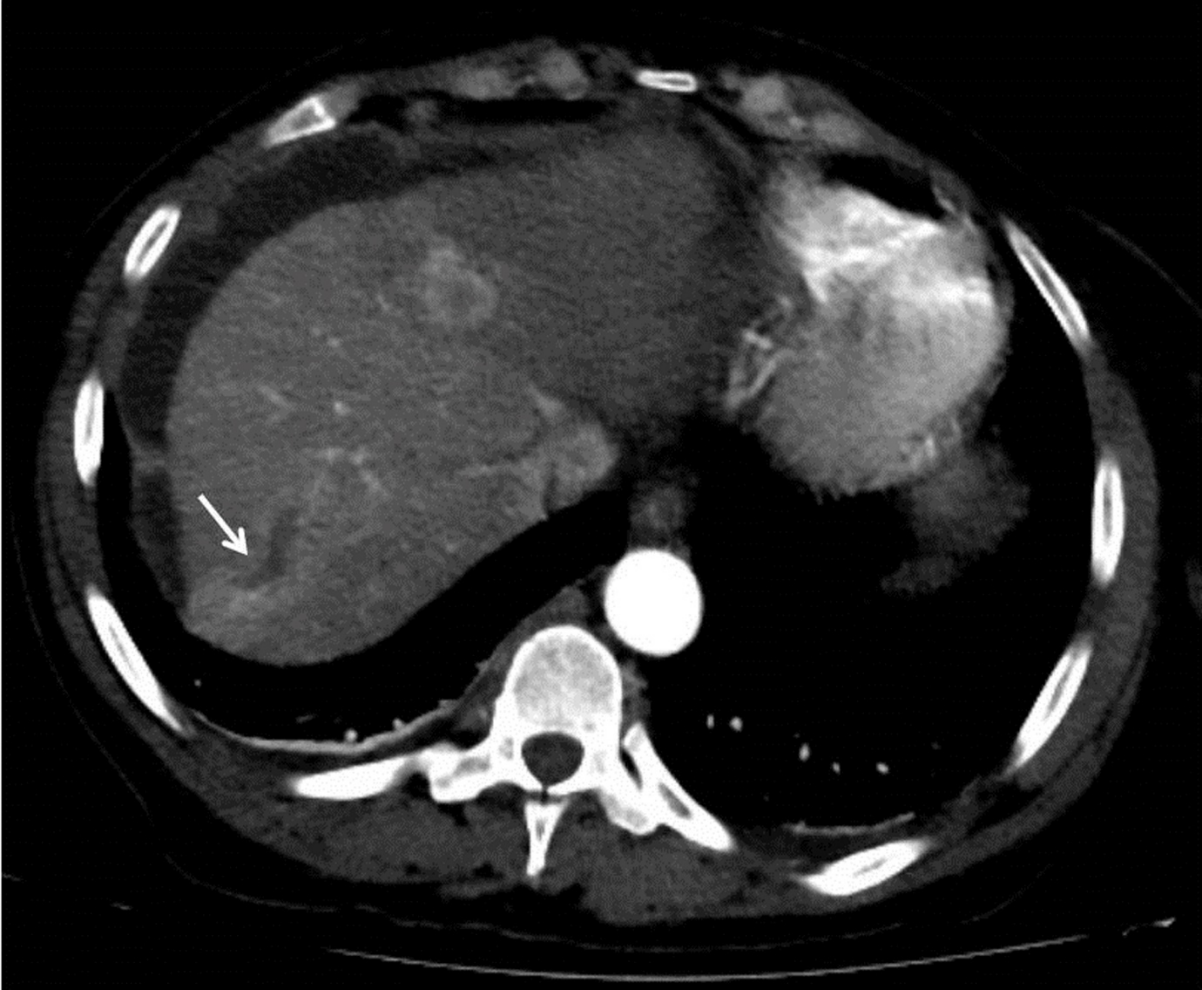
Computed tomography image of the liver with contrast showing about 4 x 2 cm-sized right main portal vein thrombosis (white thin arrow).
